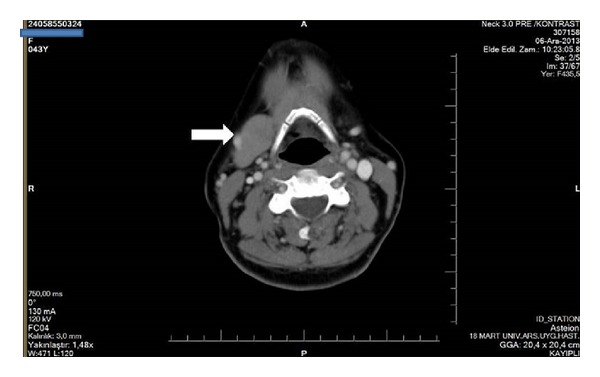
Noncontrast computed tomography axial sections demonstrate absence of left submandibular gland in the second case. White arrow shows the right submandibular gland.
